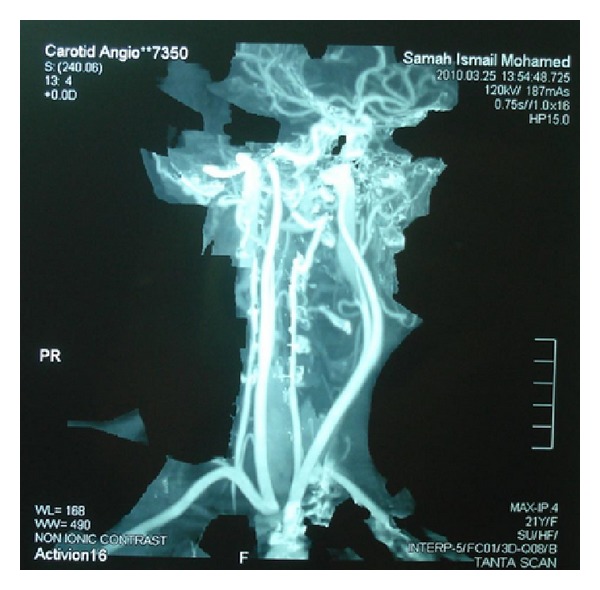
Coronal view of CT angiography showing left parapharyngeal and pharyngeal mainly hypodense, peripherally hyperdense well-defined lesion (7 × 4 × 3 cm) indenting the left pharyngeal wall but not infiltrating it.
